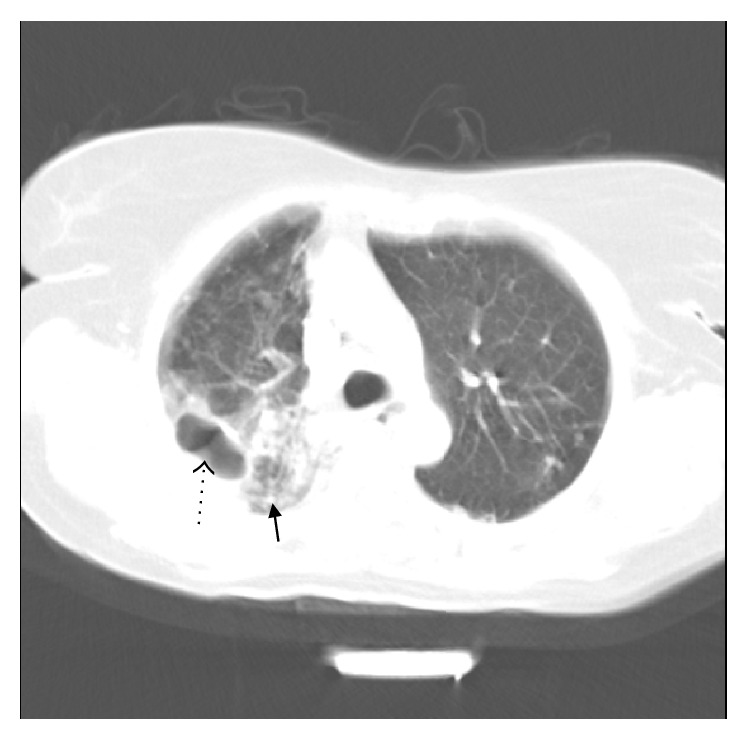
66-year-old female. Spiral CT scan below the level of left pulmonary artery (lung window). There is cavitary consolidation in posterior segment of right upper lobe (dotted arrow) along with adjacent nodular infiltration (thick arrow).
